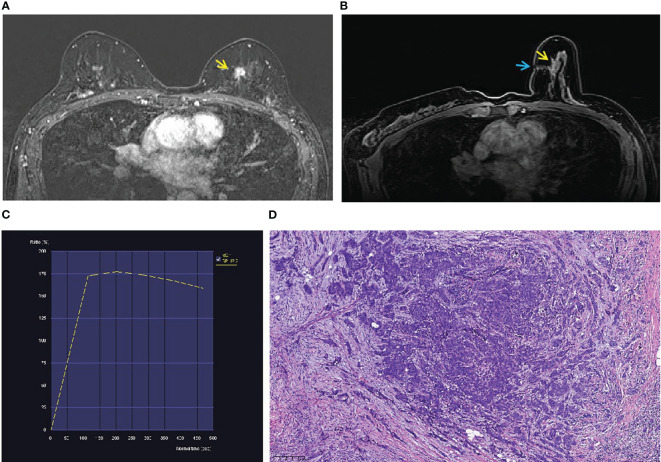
A 52-year-old woman with a family history of breast cancer. (A) An irregular mass (yellow arrow) located in the inner quadrant of the left breast (BI-RADS 4B) was shown in T1-weighted fat-suppressed contrast-enhanced MRI. It was not palpated and detected by ultrasound or mammography. (B) The mass (yellow arrow) was performed by MR-guided wire localization (blue arrow). (C) The dynamic contrast-enhanced time–signal intensity curve (TIC) appeared as “wash-out type”. (D) The lesion was confirmed as invasive carcinoma (grade II).
